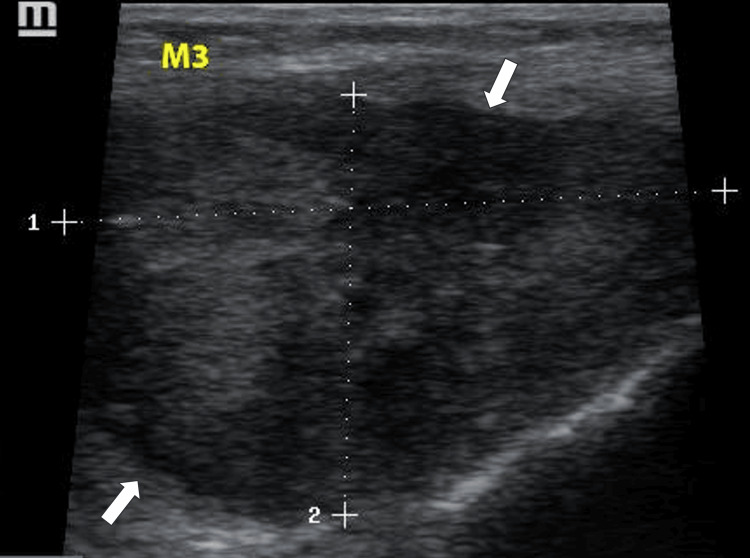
Ultrasound image of M3 The image shows a mass located in the suprapatellar compartment behind the tendon sheath of the vastus lateralis, measuring 4.56 cm (dotted line 1) x 2.80 cm (dotted line 2)
